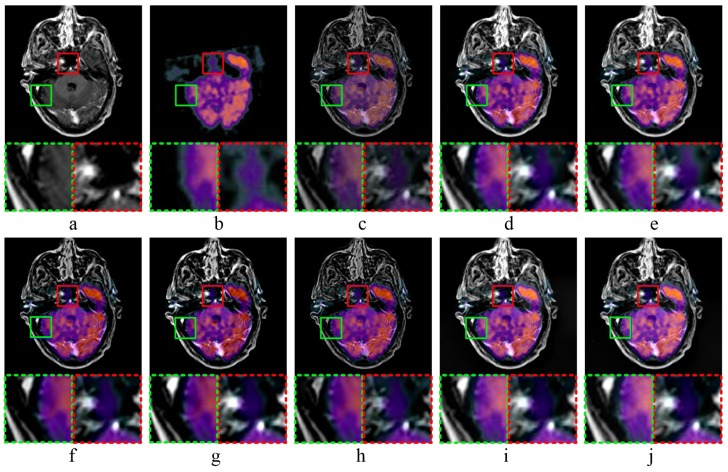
MR-PET image fusion Experiment 2: (a,b) source images; and (c–j) the fused image obtained by MST-SR, NSCT-PC, NSST-PCNN, ASR, CT, KIM, CNN-LIU, and the proposed method, respectively. Two partially enlarged images marked in green and red dashed frames correspond to the regions surrounded by green and red frames in the fused image.
